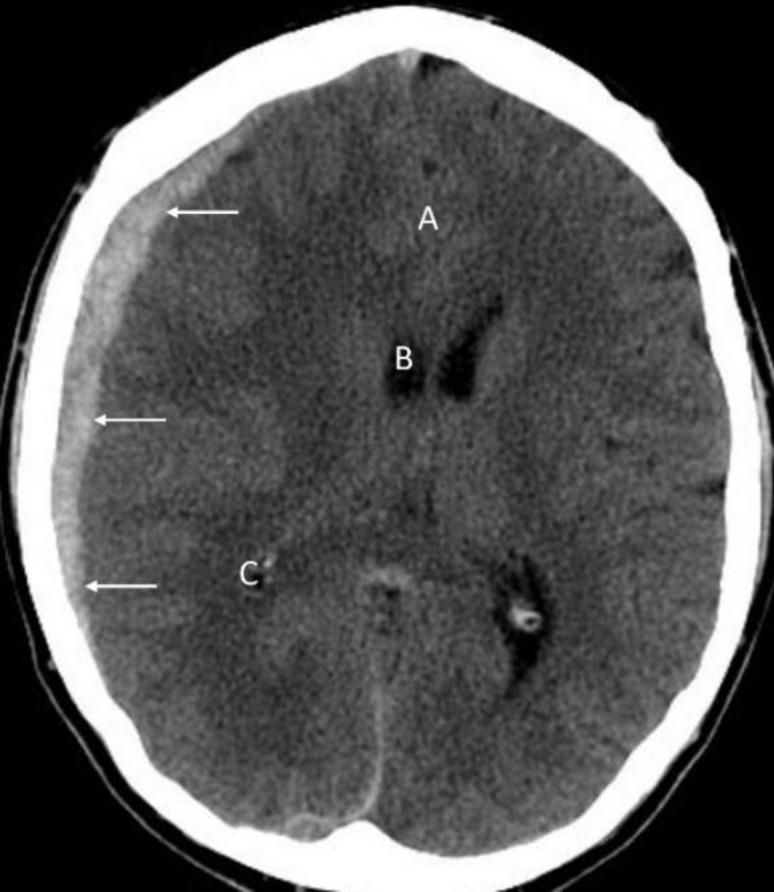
Non-contrast axial CT brain of a 31 years-old male with an acute subdural hematoma (arrows) following a road traffic accident. Note the midline shift (A) and compression of the frontal (B) and occipital (C) horns of the lateral ventricle suggesting mass effect
